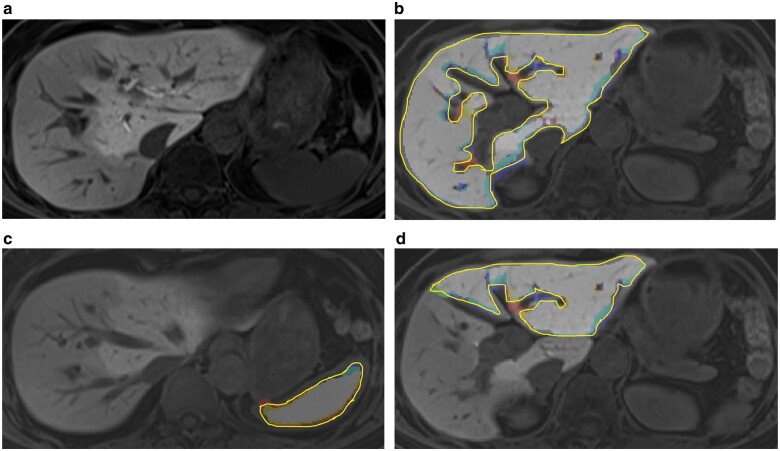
Example of gadoxetate disodium-enhanced MRI and segmentation in a patient with hilar cholangiocarcinoma located in the right hepatic duct with unilateral biliary drainage of the left liver and preoperative portal vein embolization a Original gadoxetate disodium-enhanced MRI (EOB-MRI)); b segmented whole liver; c segmented spleen; and d segmented future remnant liver (FLR) after right hemihepatectomy.
